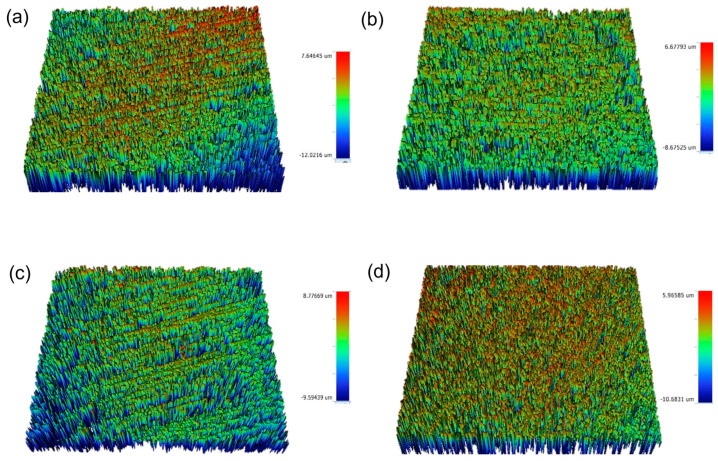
Surface roughness (Ra) of manual metal scaling of each: (a) CD, (b) CT, (c) IPE, and (d) IPS. CD; zirconia-reinforced lithium silicate (Celtra Duo). CT; advanced lithium disilicate (CEREC Tessera). IPE; lithium disilicate (IPS Emax CAD). IPS; leucite-reinforced (IPS Empress CAD).
